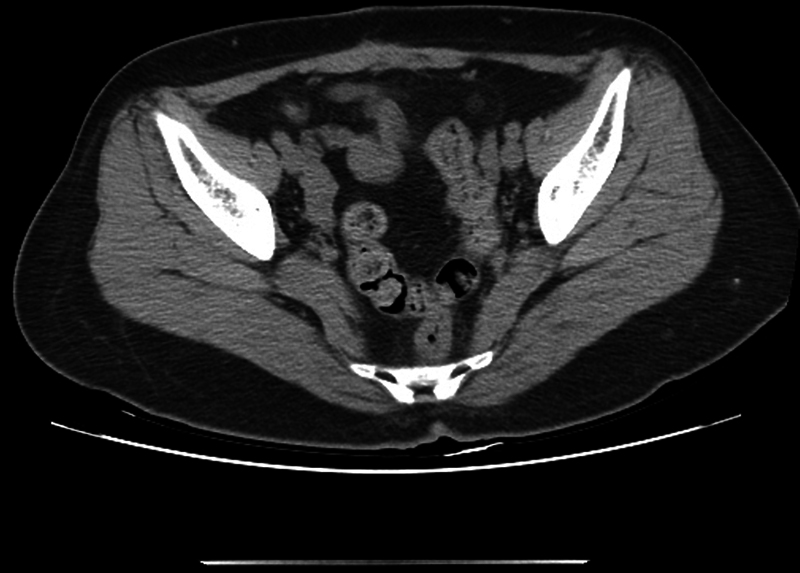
Axial contrast-enhanced CT image of case 3. Postoperative 12 months. CT, computerized tomography.
